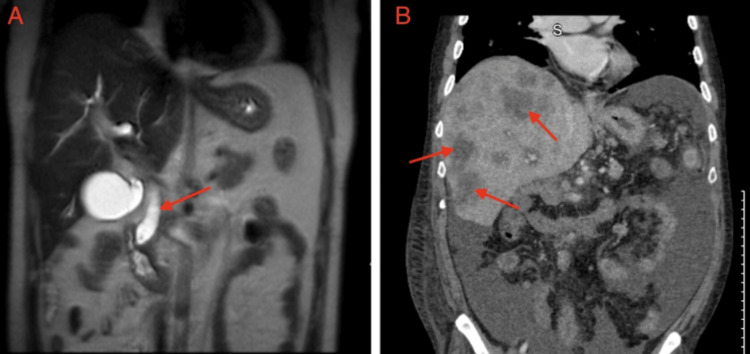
(A) MRCP showing common bile duct dilatation (red arrow). (B) Computed tomography of the abdomen and pelvis showing metastatic liver lesions (red arrows). MRCP, magnetic resonance cholangiopancreatography
